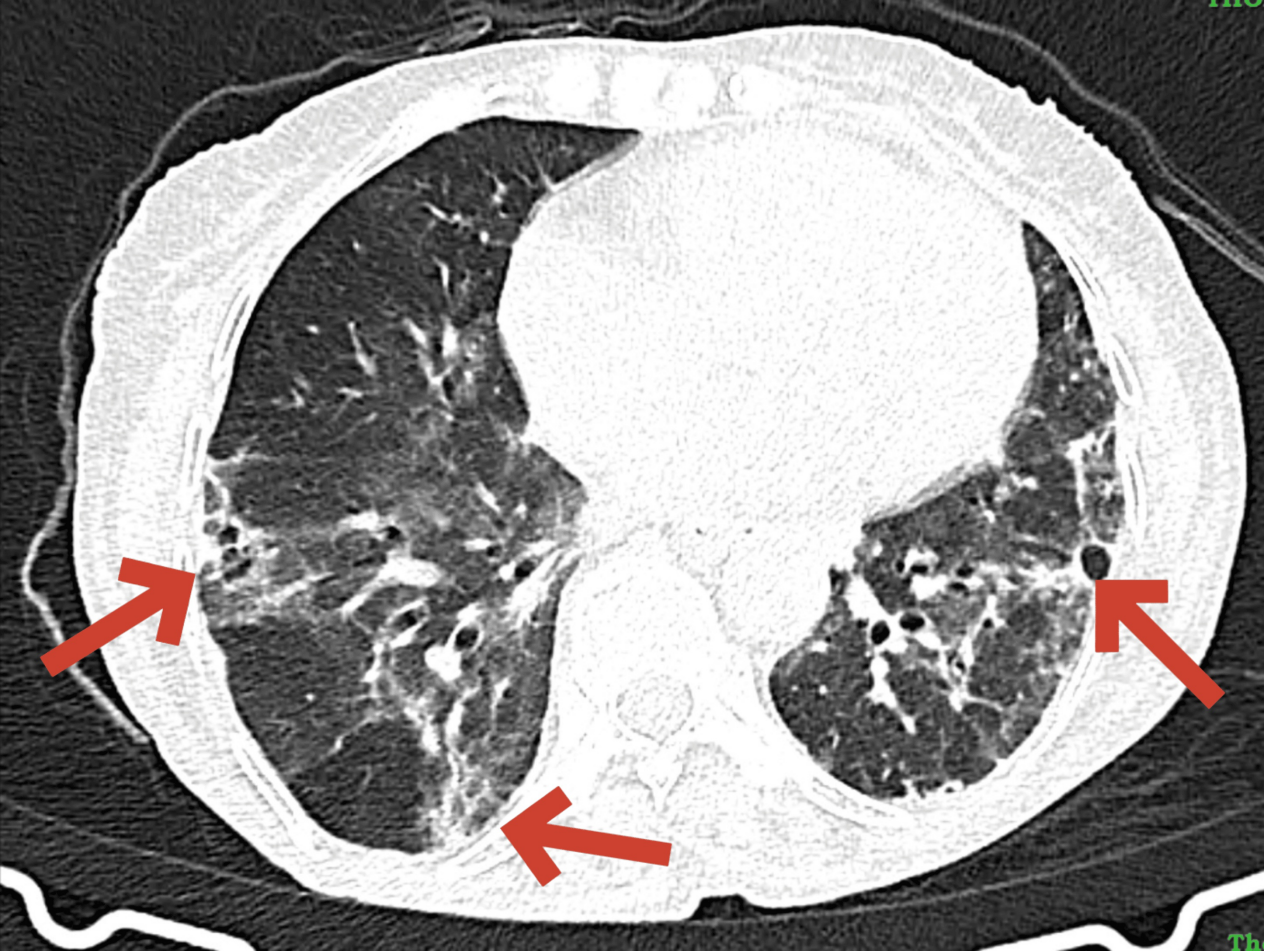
Coronal CT thorax showing lung involvement CT: Computed tomograhy The coronal CT image demonstrates significant lung involvement characterized by areas of ground-glass opacities and tractional bronchiectasis as indicated by the red arrows. These findings are consistent with interstitial lung disease likely secondary to the patient's overlap syndrome
